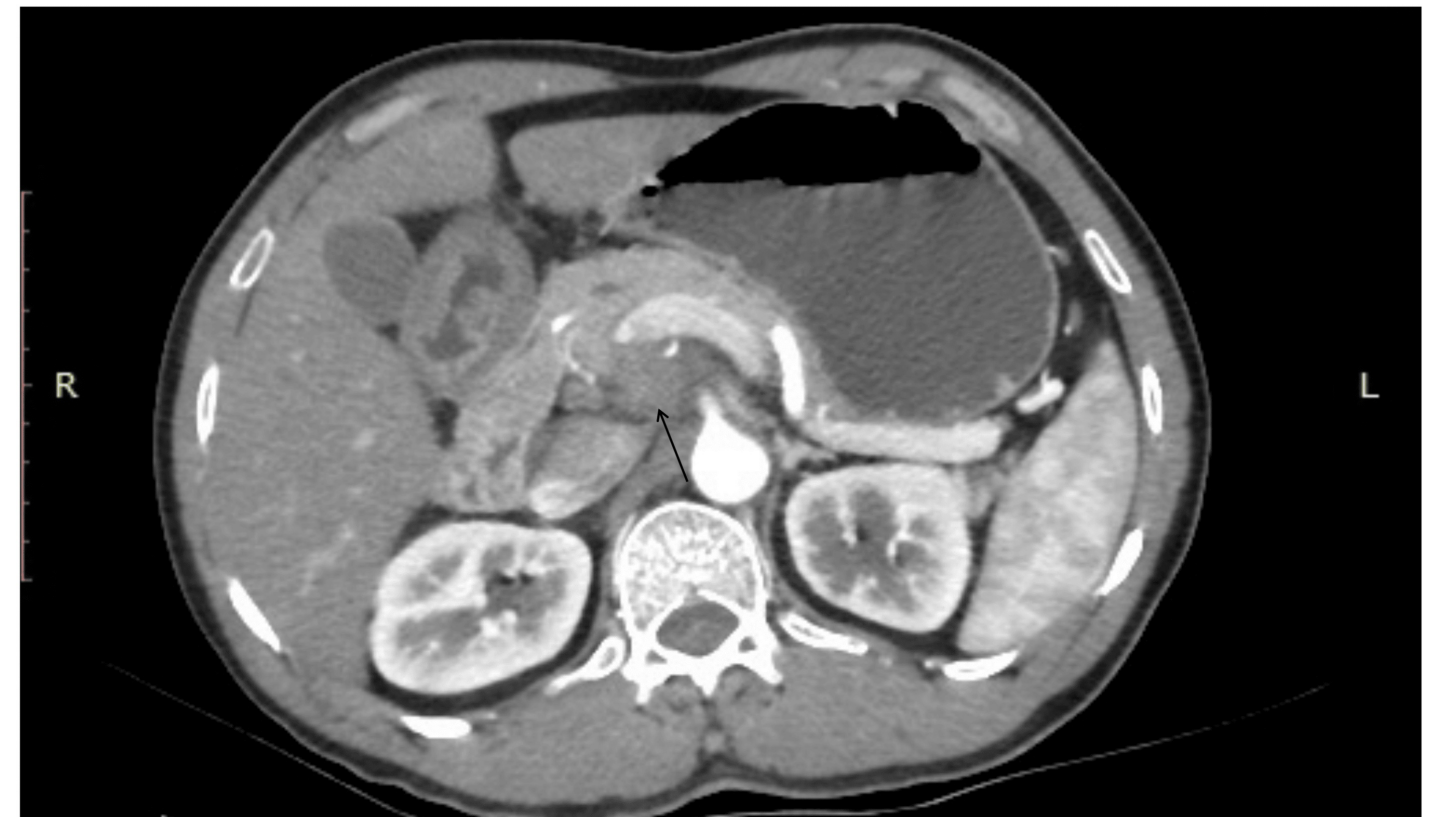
Contrast-enhanced CT of the abdomen. Ill-defined hypo-enhancing focal area in the uncinate region and enlarged celiac axis lymph nodes (arrow).
